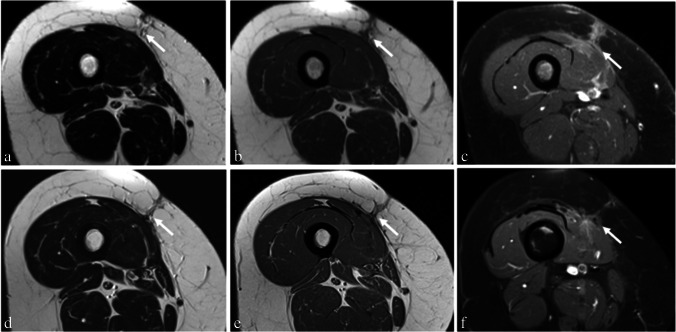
Patient example without LR in synovial sarcoma of the right thigh after multimodal therapy. Images of a 45-year-old female patient six (a–c) and 31 months (d–f) after multimodal therapy for a synovial sarcoma of the right thigh without signs of LR in further follow-up. a T2-weighted image shows slight diffuse increased signal intensity in the area of resection (arrow) while slight T1-hypointensity in subcutaneous fat was evaluated (b). Also, marked diffuse contrast uptake is shown in the area of resection (c). In a T2-weighted image 36 months after therapy (d), slight diffuse edema is present, while marked contrast uptake (arrow) in the area of resection is present (f). Minimal T1-hypointensity was detected in the area of resection (e)
